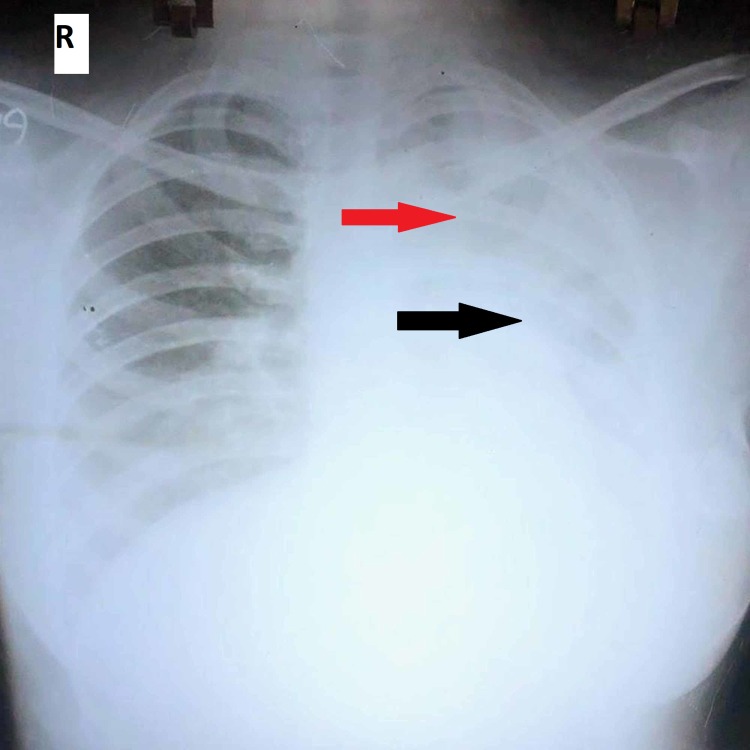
Chest radiograph on admission showing left upper (red arrow) and mid-zone (black arrow) heterogenous opacities with air bronchogram.
